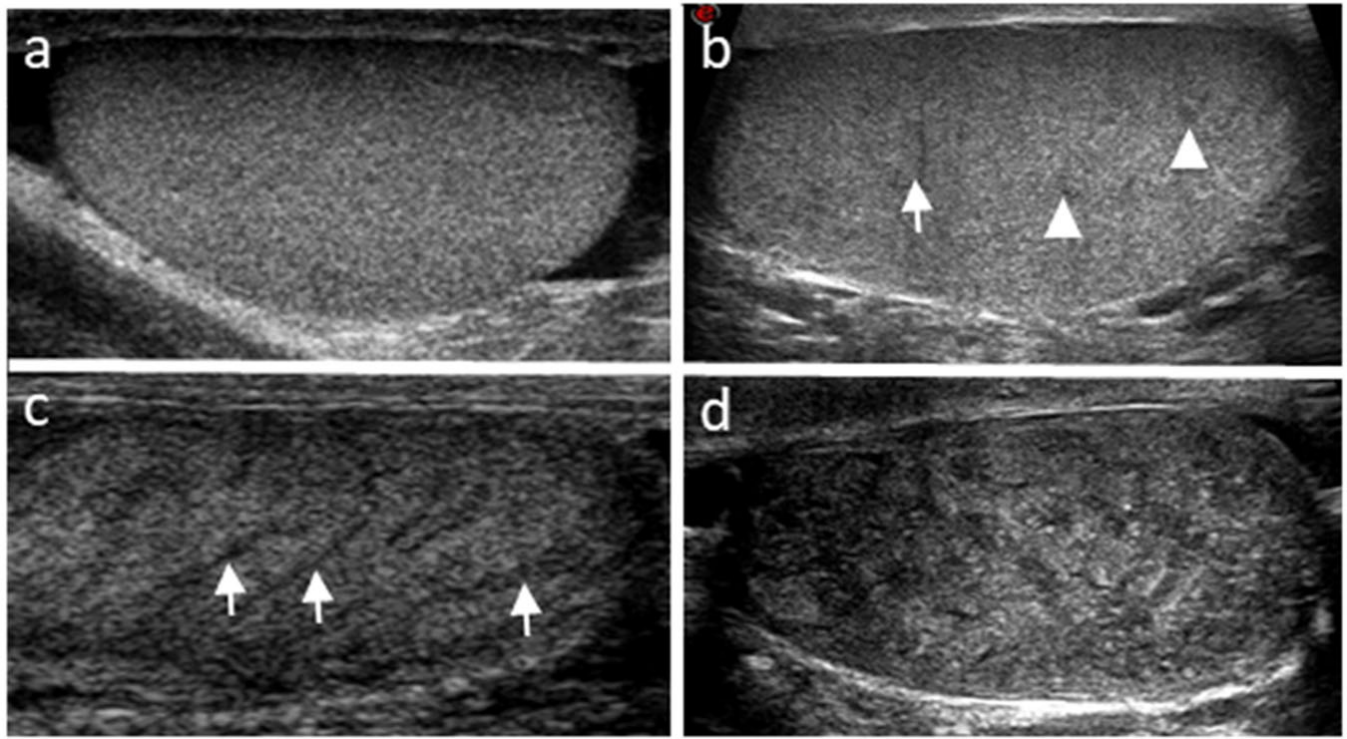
Testicular echotexture homogeneity classification of the EAA ultrasound consortium. a Homogeneity; b mild (grade 1) inhomogeneity: the presence of small hypoechoic foci (arrowheads)/thin hypoechoic striae (arrows); c moderate (grade 2) inhomogeneity: the presence of thick hypoechoic striae (arrows); d severe (grade 3) inhomogeneity: diffuse inhomogeneity with “netting”/“geographical map” appearance. Adapted from reference [4]
